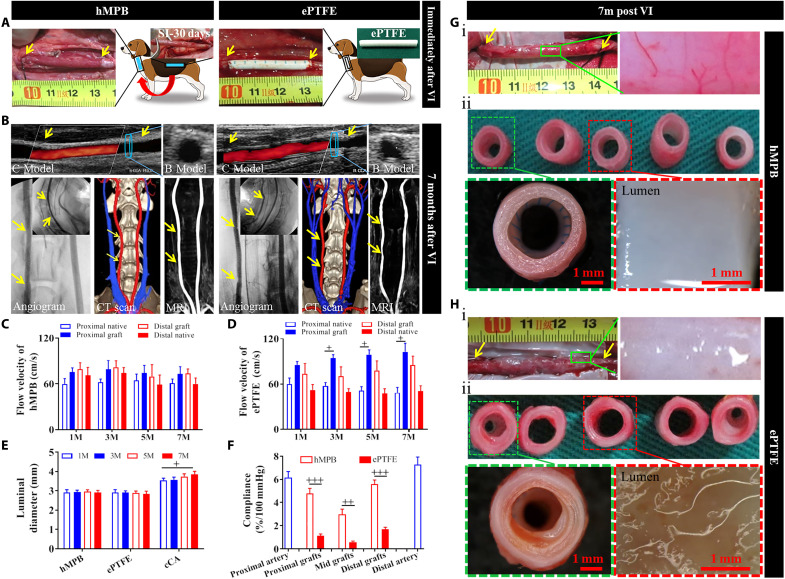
hMPB and ePTFE graft implantation in cCA replacement model. (A) hMPB and ePTFE graft images before VI and immediately after VI (*n* = 3). (B) Representative CDU, angiography, CT 3D reconstruction, and MRI images of hMPB and ePTFE grafts at 7 months after VI (yellow arrows indicate suture sites). (C to E) Ultrasound-based measurement of flow velocity (C and D) and luminal diameter (E) of hMPB and ePTFE grafts after VI (*n* = 3). Statistical significance was calculated by two-way ANOVA with Tukey’s test. Symbol (+) indicates the comparison in the same group, ^+^*P* < 0.05. (F) Quantification of in vivo compliance of the hMPB-V7m and ePTFE-V7m as well as their adjacent proximal and distal cCA (*n* = 3). Statistical significance was calculated by two-way ANOVA with Tukey’s test. Symbol (+) indicates the comparison in the same group, ^++^*P* < 0.01, ^+++^*P* < 0.001. (G) hMPB-V7m and (H) ePTFE-V7m exterior view (i) and five cross-sectional pieces (ii). Green boxes indicate zoomed exterior views. Green dashed boxes indicate sutured end and magnified view. Red dashed boxes indicate the pieces taken from the middle of the graft for observation of graft lumens.
